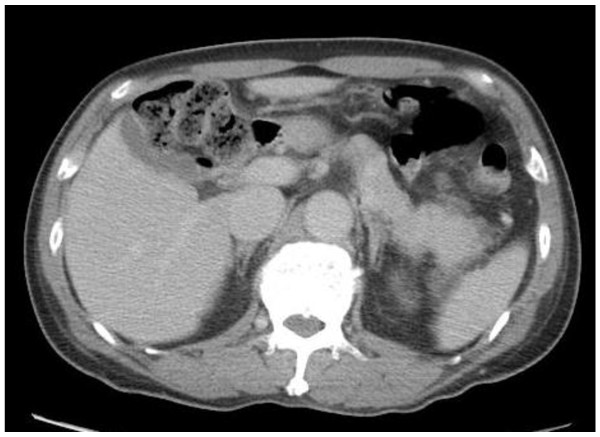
Abdominal computed tomography (CT) on day 5. Abdominal CT to investigate the cause of epigastric pain showed localized swelling of the pancreatic body and tail, with no cholelithiasis or tumor obstructing the common bile duct or pancreatic duct, suggesting idiopathic pancreatitis.
